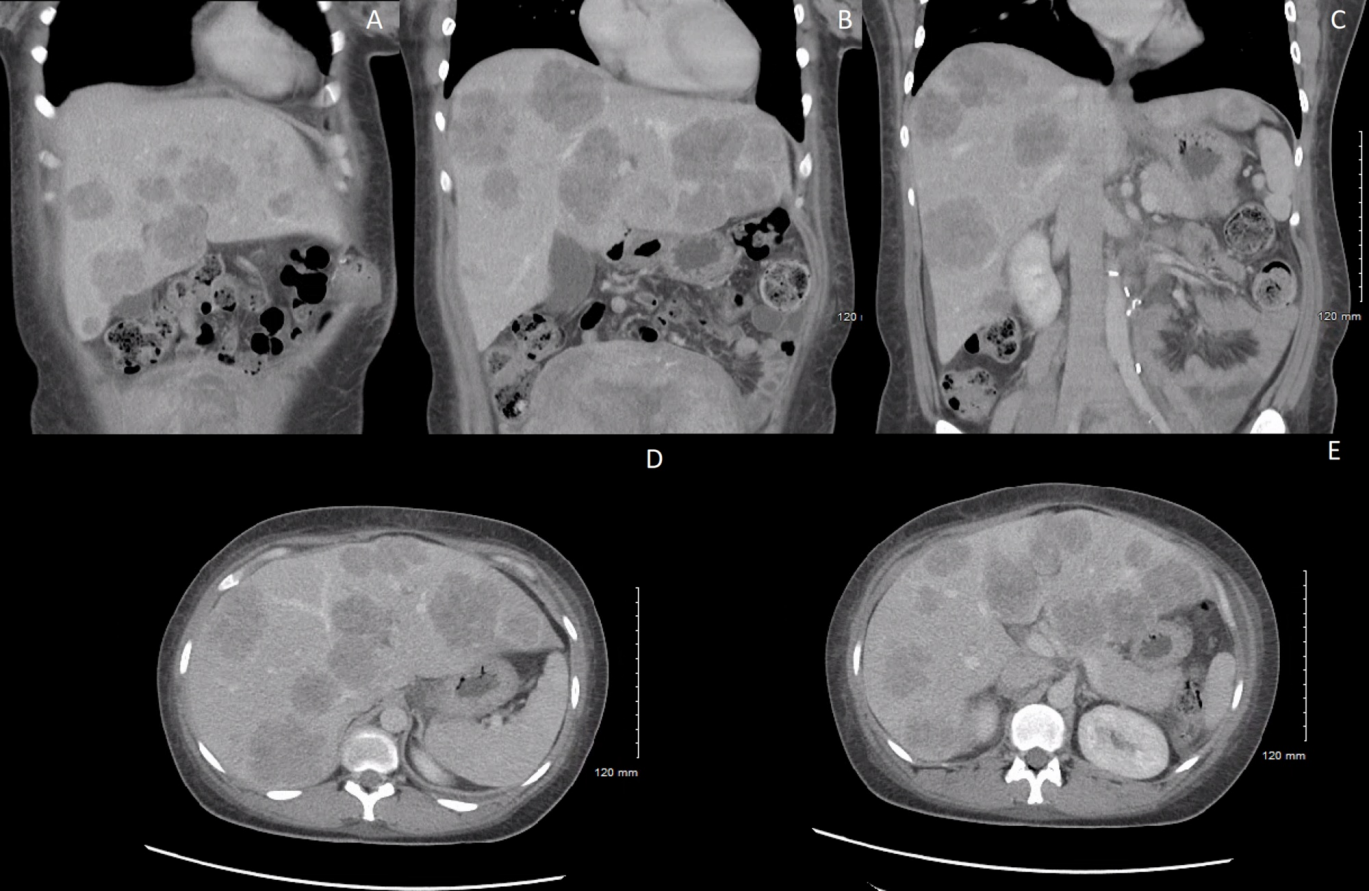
Computed tomography imaging (A-E) Multiple diffuse hepatic lesions representing metastasis
